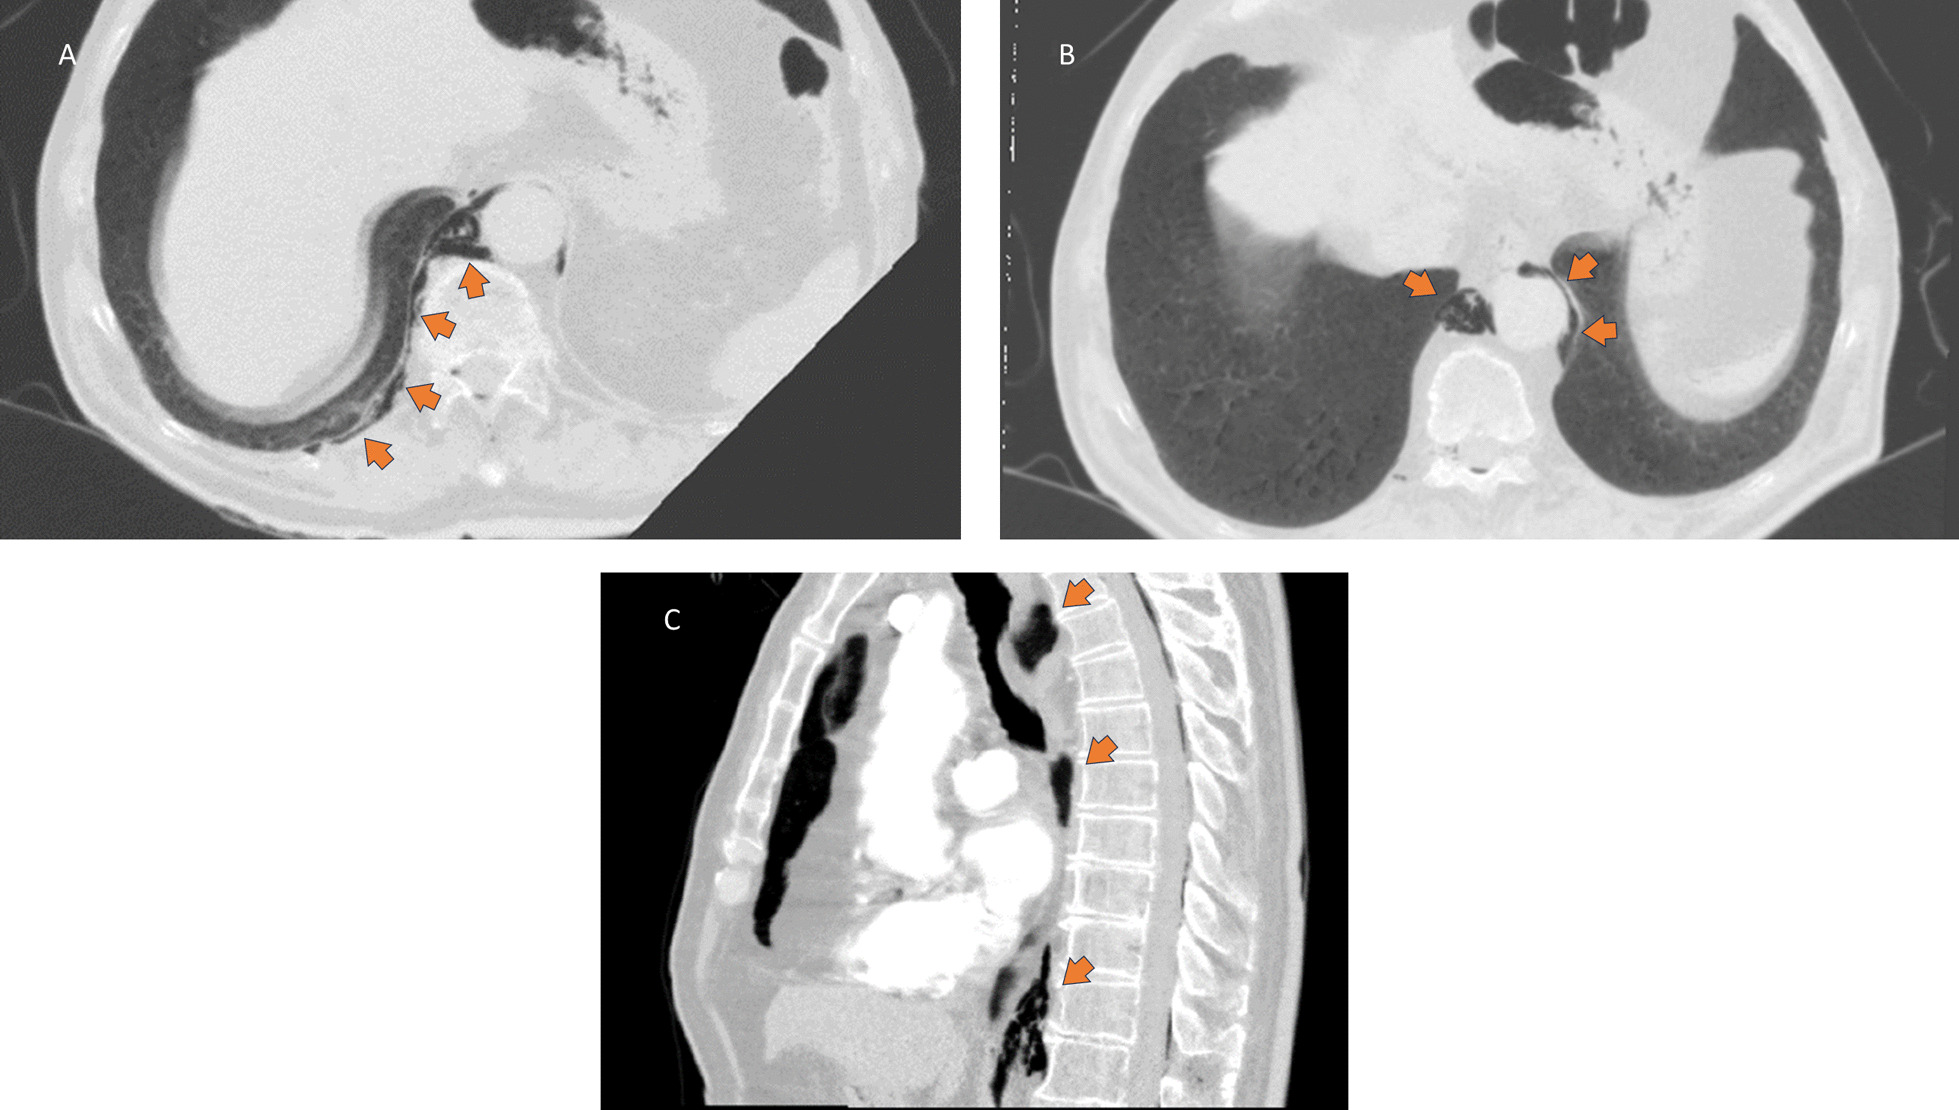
CT Scan of the abdomen/chest A Free air (arrows) in the paravertebral subcutaneous tissue and around the aorta (demonstration of tissue continuation); B Free air (arrows) present in the mediastinum (pneumomediastinum) surrounding the aorta; C Sagittal view (arrows show air in different levels)
